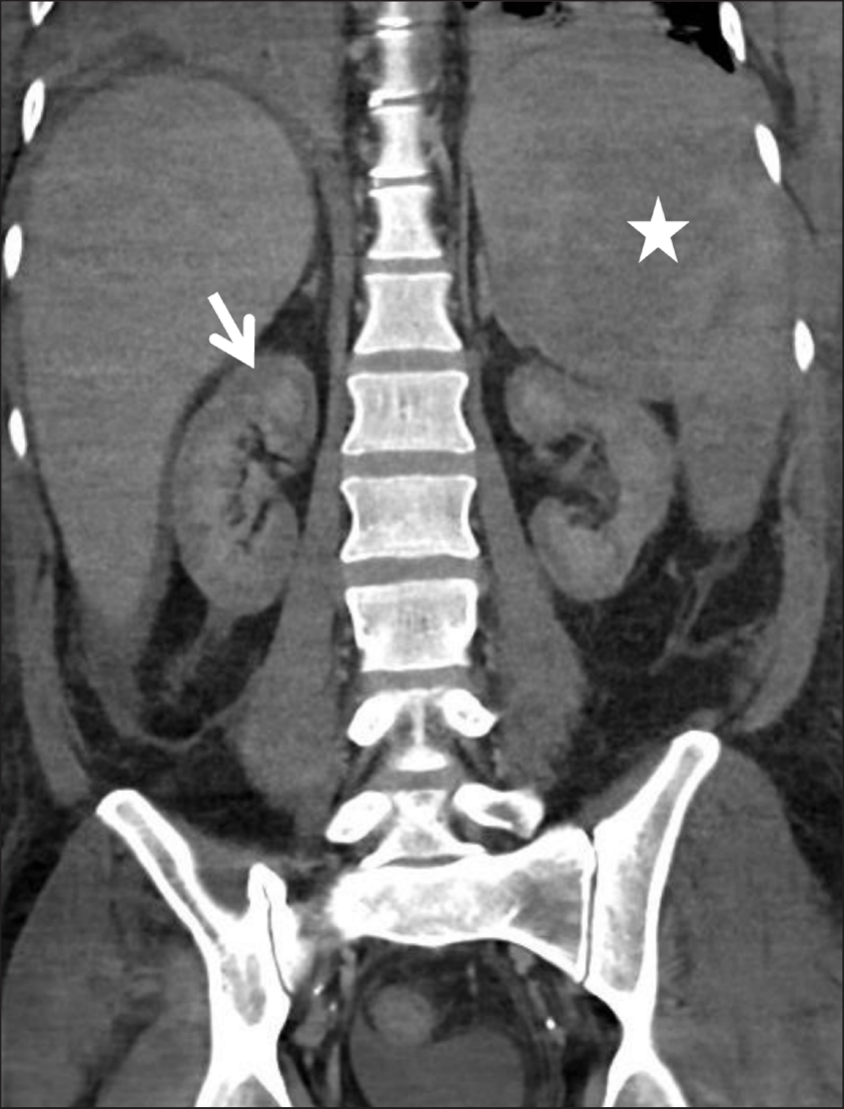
Coronal contrast-enhanced CT in the venous phase shows non enhancing renal cortex (white arrow). Also note splenomegaly (asterisk) and the small amount of perihepatic ascites.
